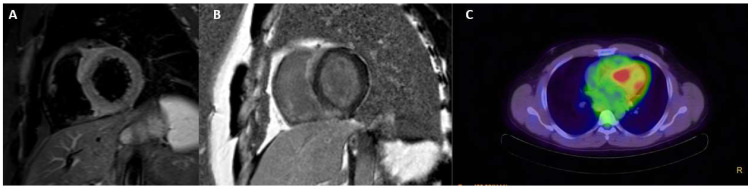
Cardiac sarcoidosis. CMR T2 imaging showing septal oedema (A). Septal LGE (B). Corresponding FDG-PET imaging showing high septal inflammation (C).
